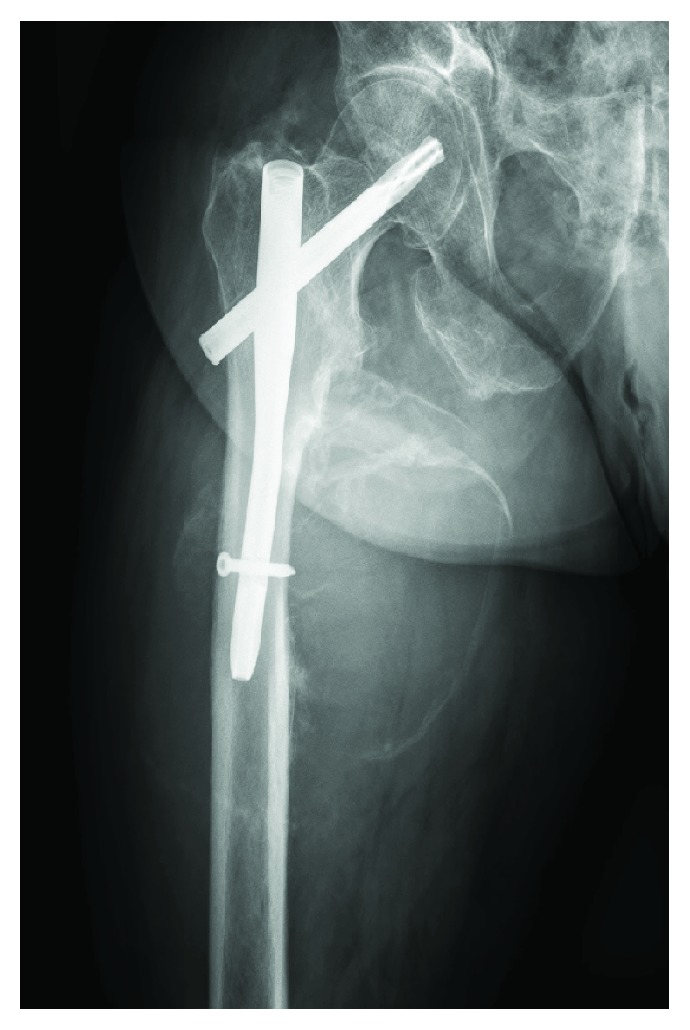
Plain X-rays eight months postoperatively showed the presence of a medial mass centred over the distal locking screw with calcification of the peripheral borders, suggestive for an (infected) hematoma. Compression and severe osteolysis of the medial border of the femur were remarked.
